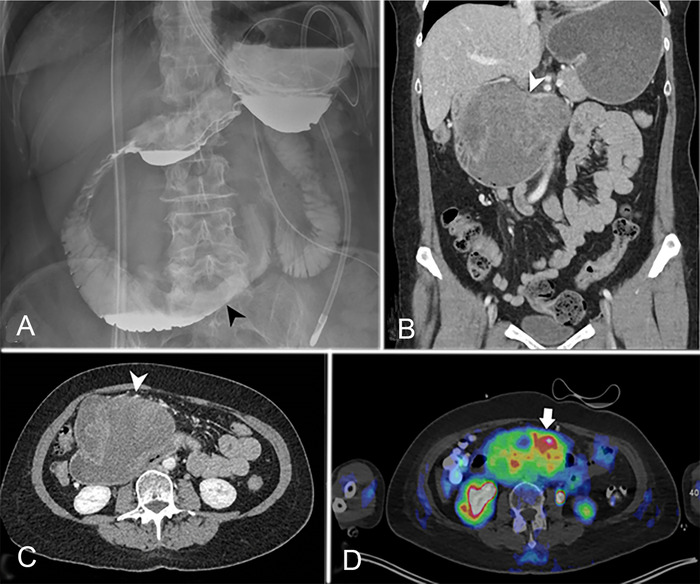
(A) Anteroposterior view of the abdomen after administration of oral contrast (Omnipaque, GE Healthcare) demonstrates narrowed third and fourth segments of the duodenum secondary to mass effect (black arrowhead). (B) Coronal and (C) axial computed tomography (CT) images demonstrate a heterogeneous mass arising from the superior and anterior walls of the third segment of the duodenum (white arrowheads). Approximately 90 mL of Visipaque 320 (GE Healthcare) was administered intravenously. (D) Fluorodeoxyglucose (F 18-FDG) positron emission tomography/CT demonstrates the same mass with intense metabolic activity (white arrow). Approximately 7.15 mCi of F 18-FDG was administered intravenously. The patient's blood glucose level just prior to imaging was 90 mg/dL. Time from F 18-FDG injection to scan was 51 minutes.
